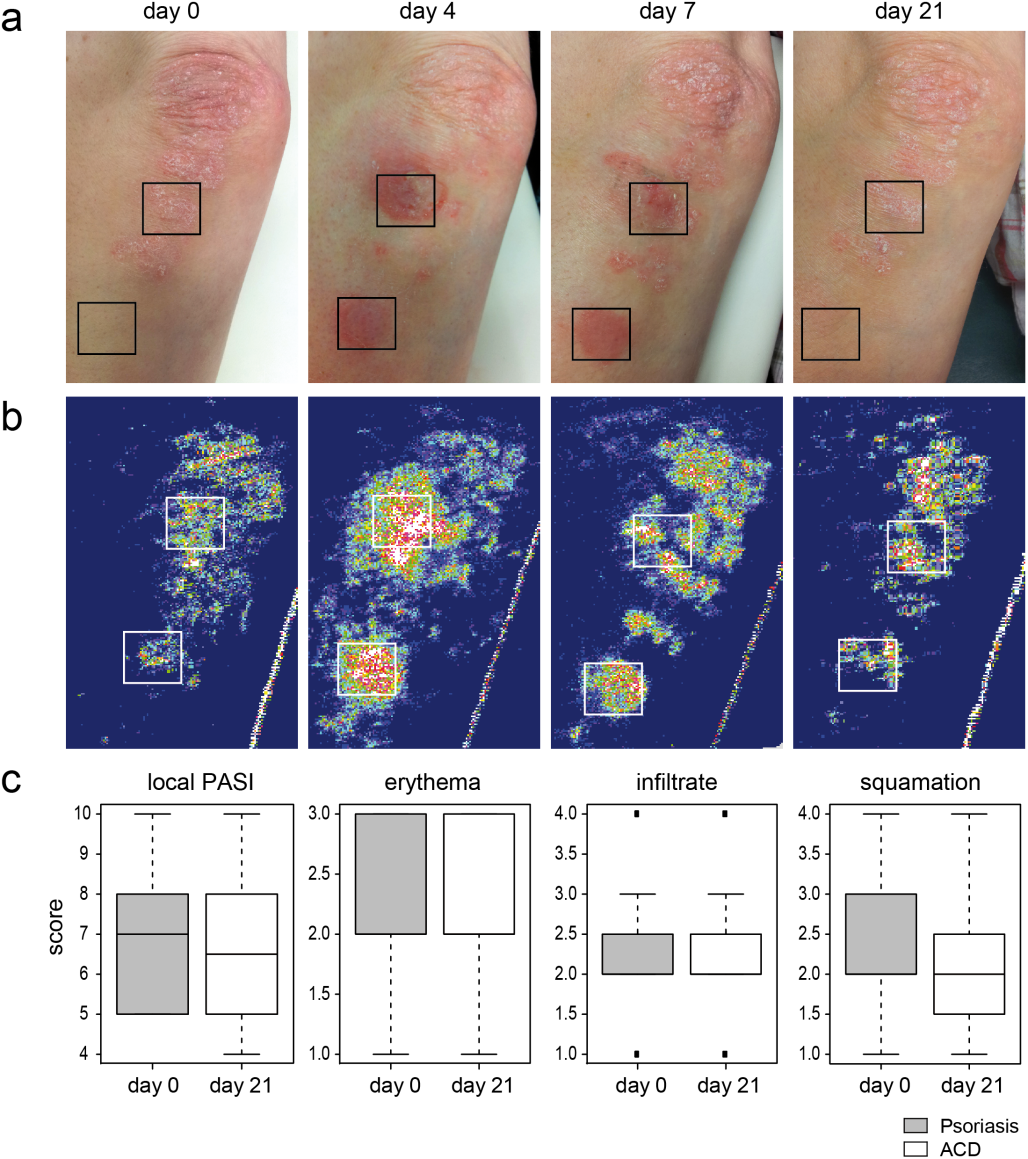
ACD and psoriasis are independent, stimulus-driven immune reactions. Nickel patch tests were used to induce ACD lesions in close proximity to or on top of a psoriasis plaque (n = 11). A Representative clinical course of psoriasis, ACD, and psoriasis/ACD mixed reaction in one patient. B Subjective clinical evaluation was confirmed investigating the local blood flow as indicator for severity of inflammation via laser doppler imaging at day 0, day 4, day 7 and day 21 (B). C Changes in local PASI, erythema, infiltrate and squamation were determined for the nickel treated psoriasis plaque before and 21 days after nickel challenge (n = 11) and presented as boxplots.
